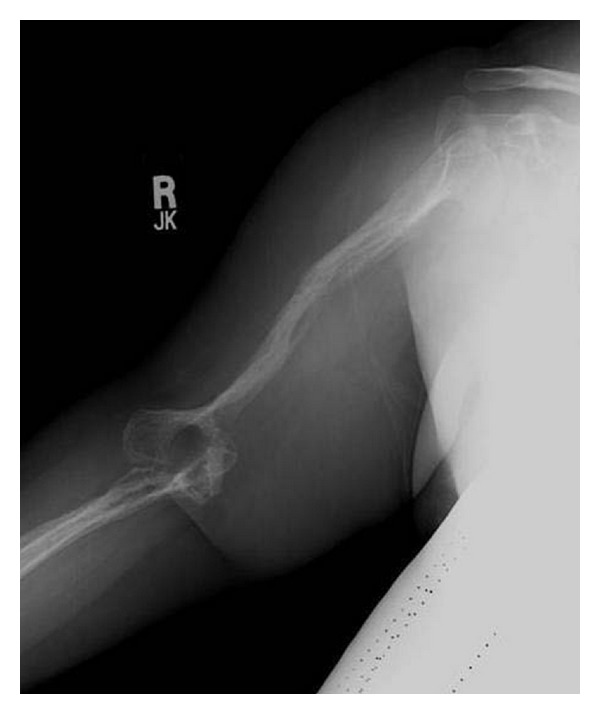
Plain radiographs of the upper extremity taken 8 years earlier revealed a destructive lytic process involving the humerus, radius, and ulna with pathologic midshaft humerus fracture and chronic dislocation of the humeroulnar joint.
